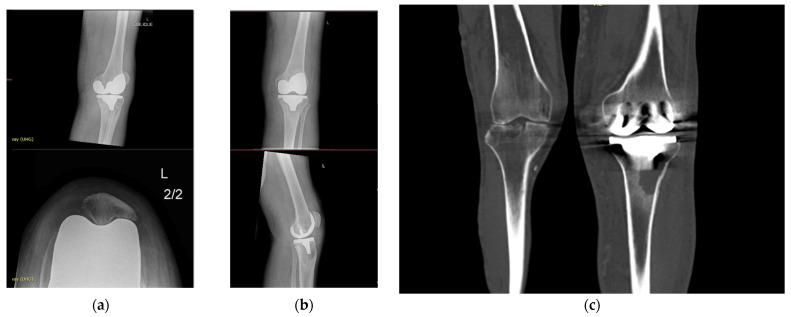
(a,b) Case 5 pre-op X-Ray. (c) CT demonstrating defect in tibia.
